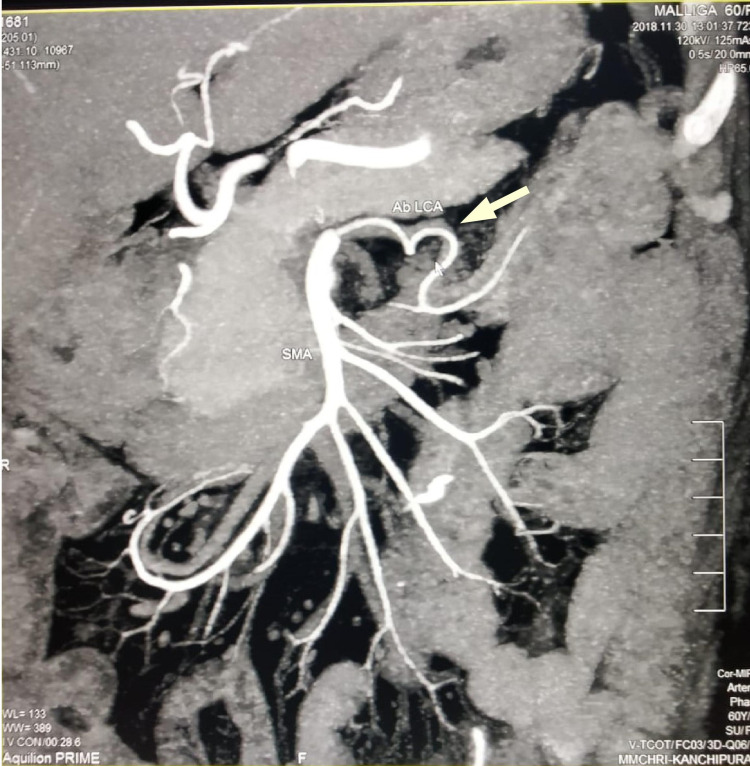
Contrast CT abdomen of ab LCA - sagittal section. Contrast CT abdomen of ab LCA - sagittal section with an arrow pointing towards it ab LCA, aberrant left colic artery
